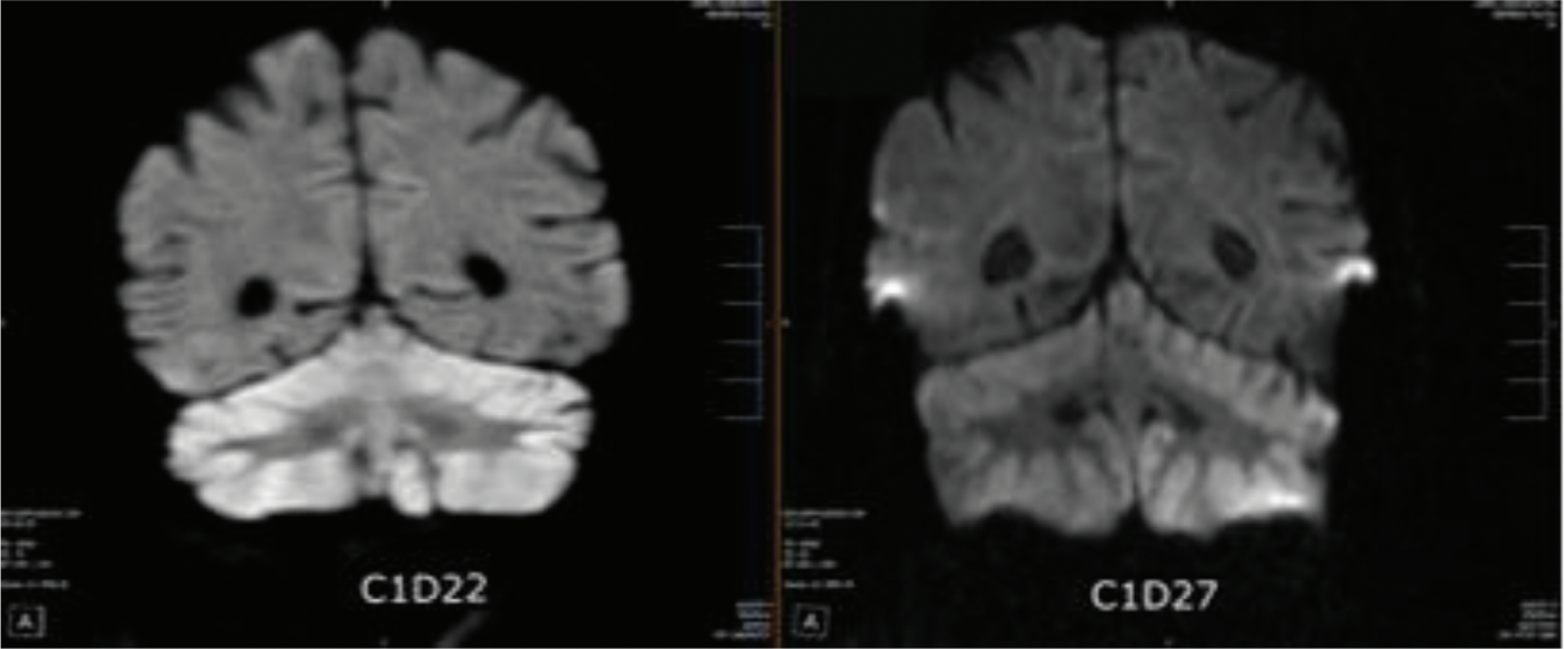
MRI brain of patient 012 with cerebellar toxicity of Minnelide. Marked cerebellar activity change is seen between days 22 (left) and 27 (right) of cycle 1.
